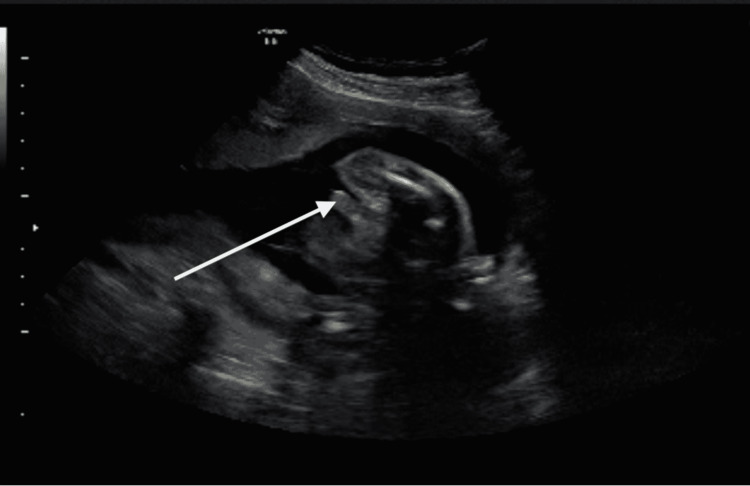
24th week ultrasound The cyst is completely resorbed
